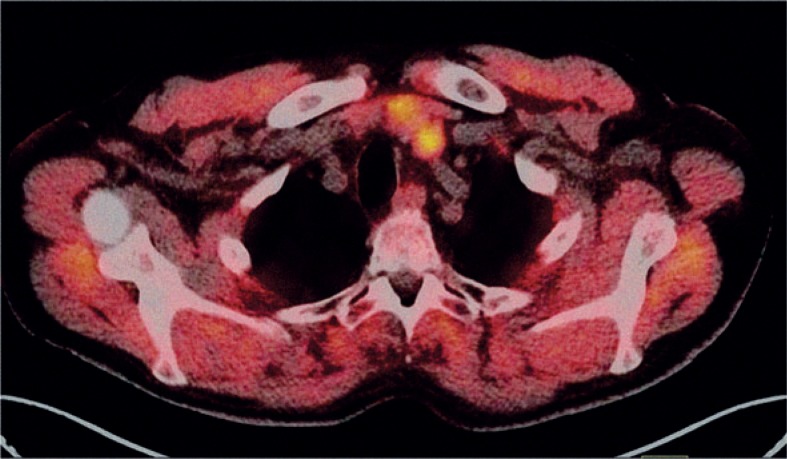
Positron emission tomography-computed tomography axial slice: Thyroid adenoma that incidentally took-up ^18^F-fluorocholine (SUVmax 3.3).
